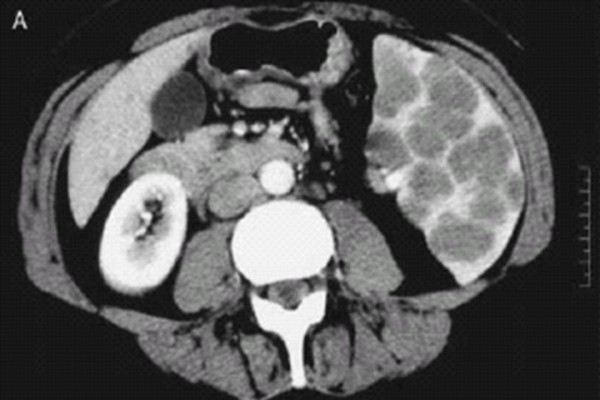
CT Image – (CT scan image showing multiple splenic nodules).
